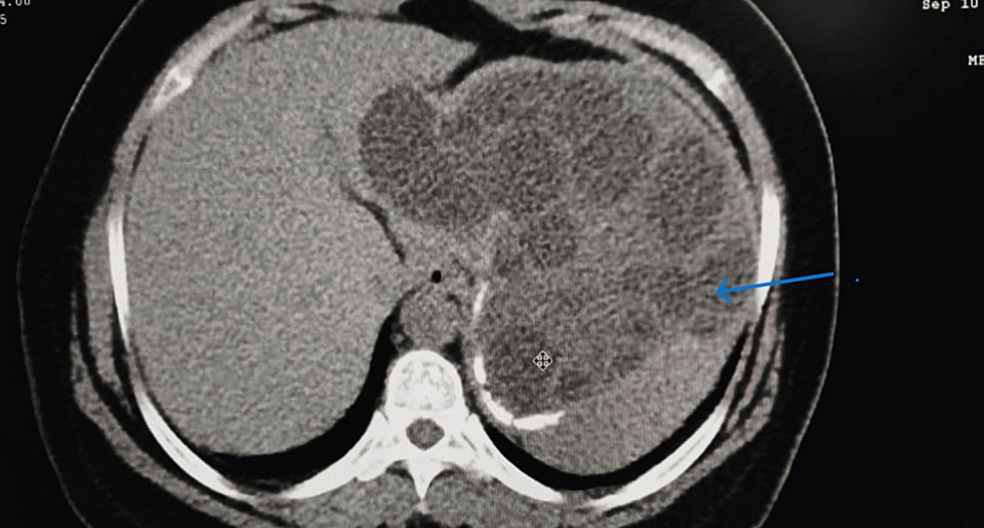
A CT scan of the abdomen showing the splenic hydatid with brood capsules (blue arrow)
